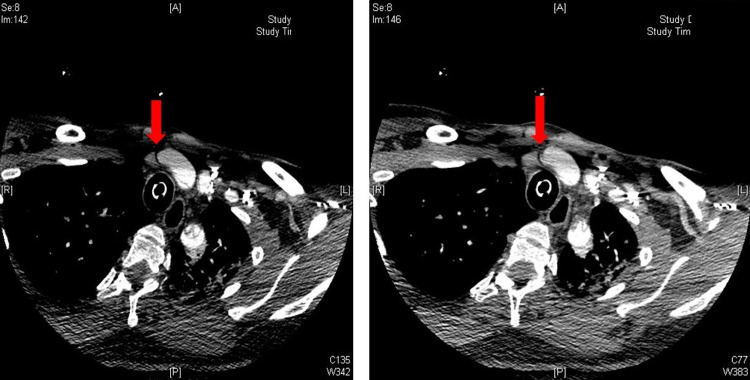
CT angiogram of the neck showing occlusion at the origin of the right common carotid artery (arrows) A: anterior, P: posterior.
